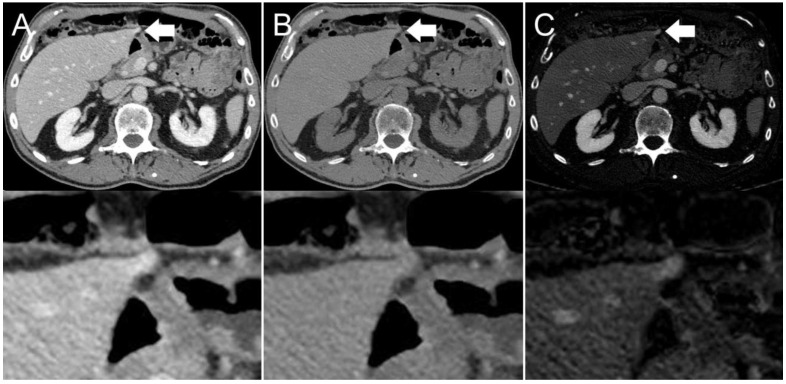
Axial contrast-enhanced CT scan of an 86-year-old man. Hyperdensity (indicated by arrows) on the left liver lobe in the (A) conventional 120 kVp image, which might be interpreted as a peristalsis-related streak artifact originating from the stomach. The (B) virtual non-contrast (VNC) image reconstruction and (C) iodine image reconstruction reveal that it is not a streak artifact, but a hyperenhancing liver lesion.
